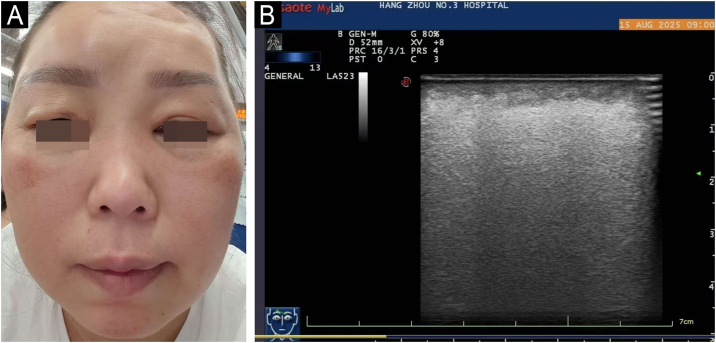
(A) Pretreatment clinical image. (B) Hyperechoic region extended approximately 20 mm above the hairline, involving the root of the nose, the temporal area and the orbits. The deep echo could not be displayed. Doppler Flow Imaging (CDFI) showed no obvious blood flow signal within it.
